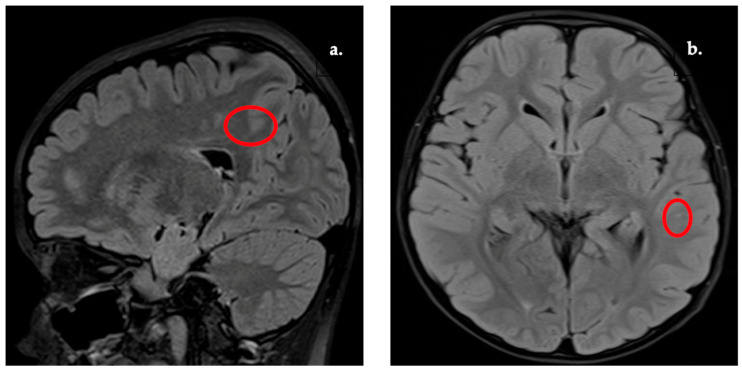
FLAIR images—WMSA in the precuneus (a), respectively, in the superior temporal gyri (b).
